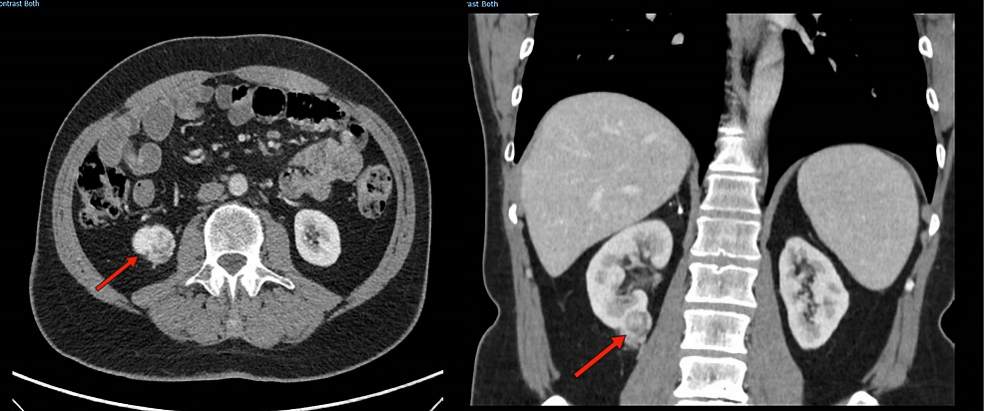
CT scan of the abdomen (axial and coronal plane) demonstrating a 2.5 cm heterogeneously enhancing lesion of the right lower renal pole as designated by the arrow. CT: computed tomography.
